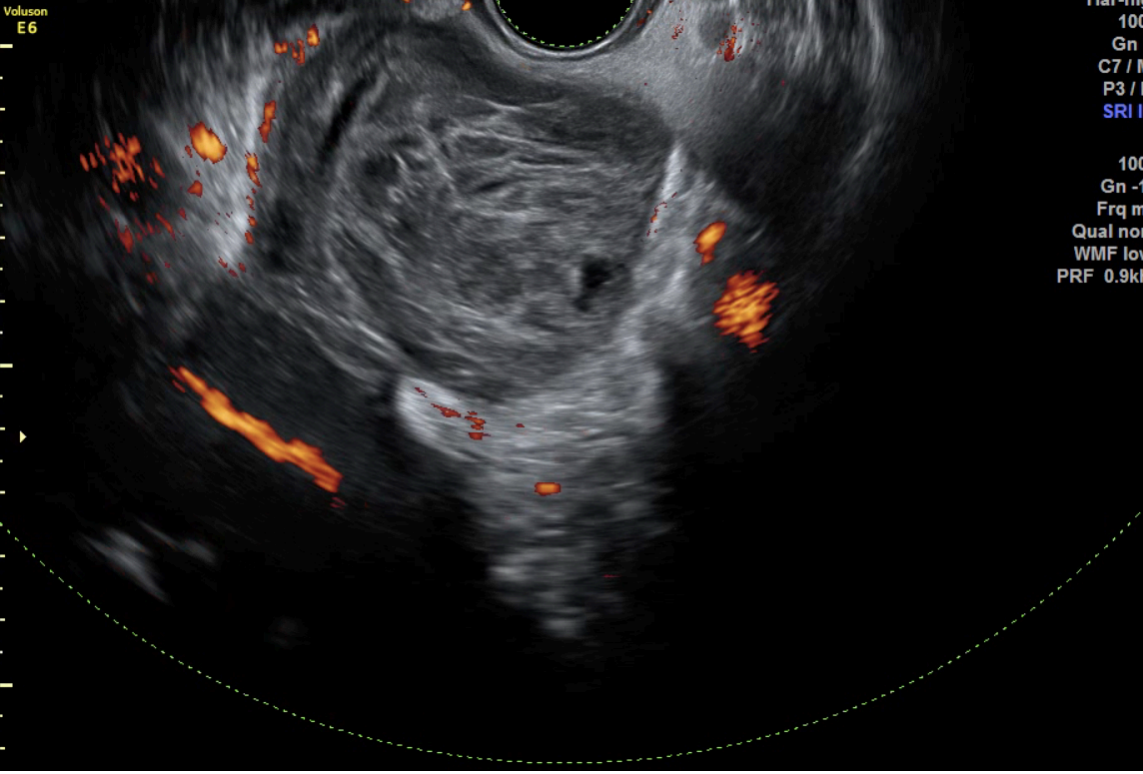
Vaginal ultrasound revealing a suspicious solid and cystic tumor possibly originating from the left ovary.
